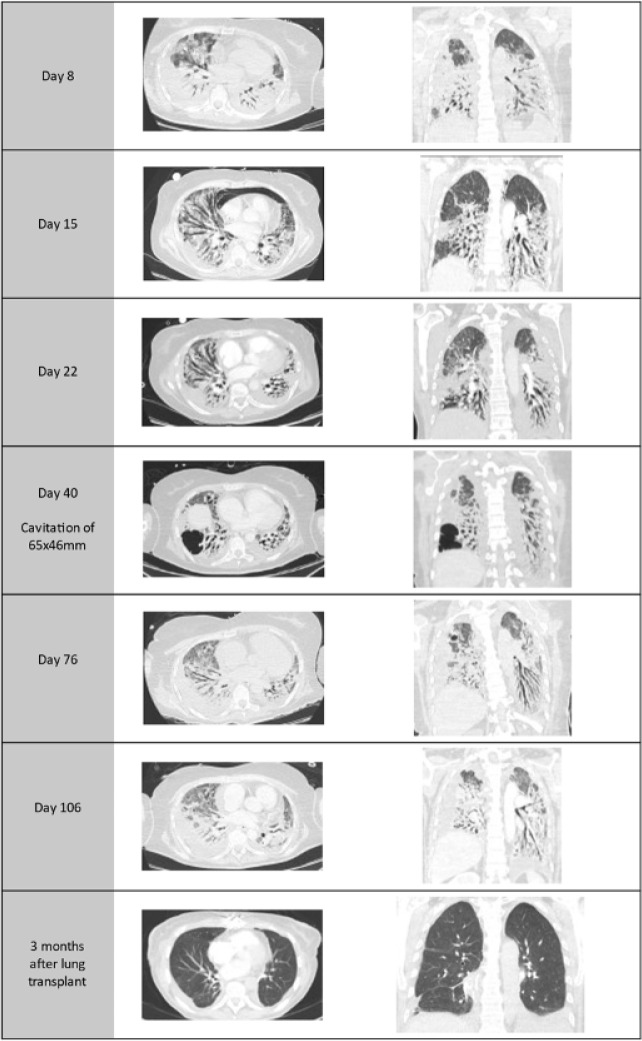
Serial thoracic computed tomography scan evaluations (days are counted
